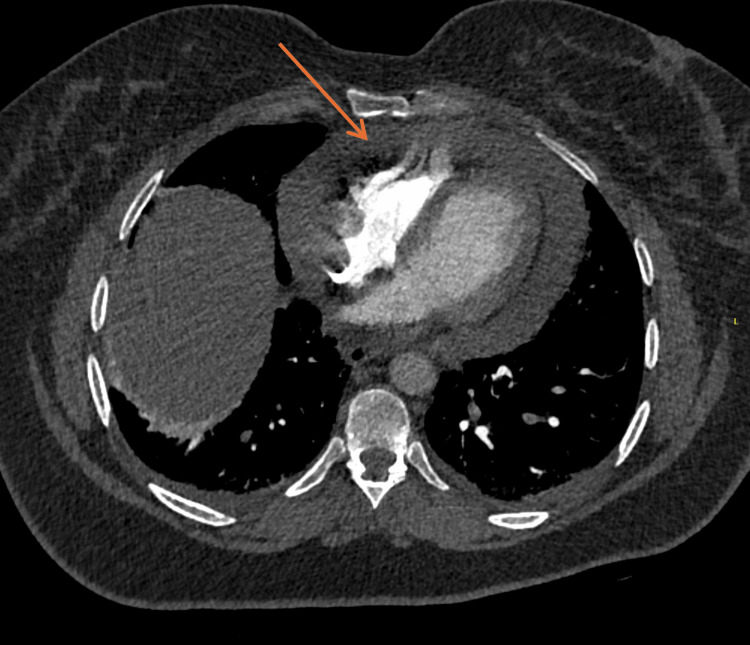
CT-pulmonary angiography demonstrating large pericardial effusion (orange arrow).
